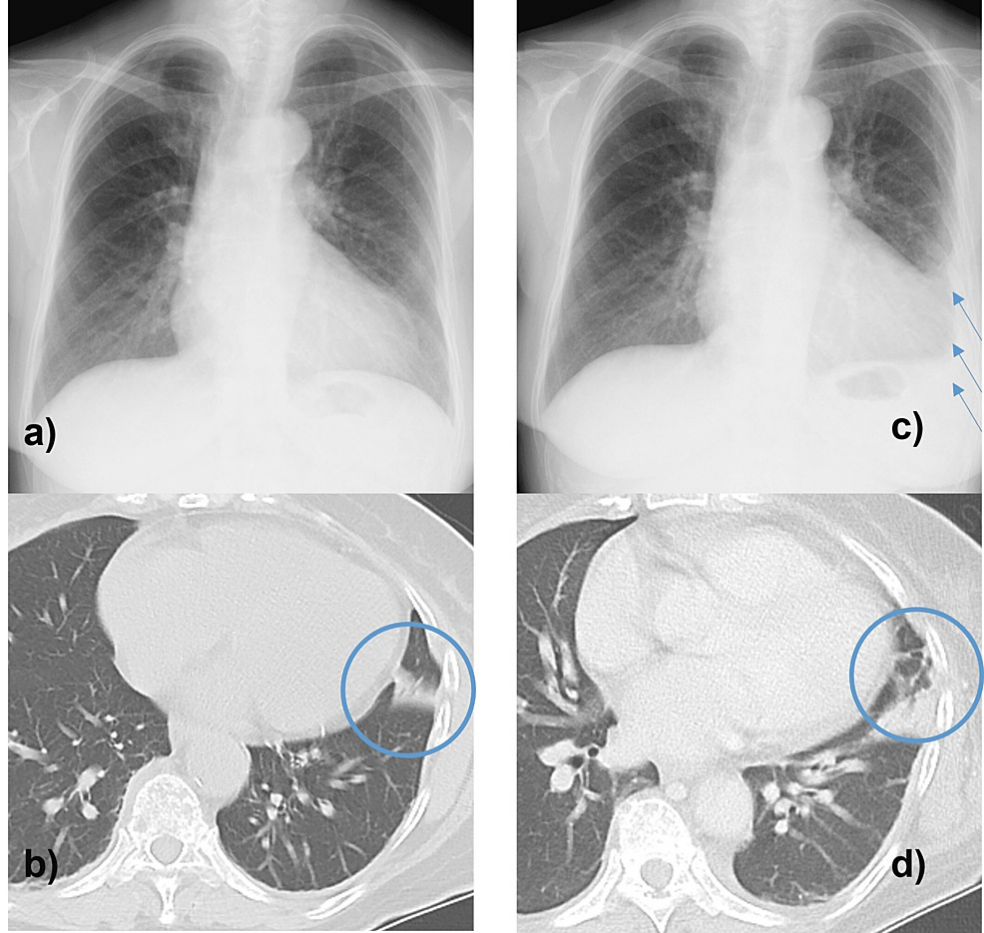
Chest X-ray and CT showing progressive infiltration shadow in S8 left lung and increasing pleural effusion a) Chest X-ray at the initial visit, b) Chest CT at the initial visit, c) Chest X-ray after 2 weeks, d) Chest CT after 2 weeks
